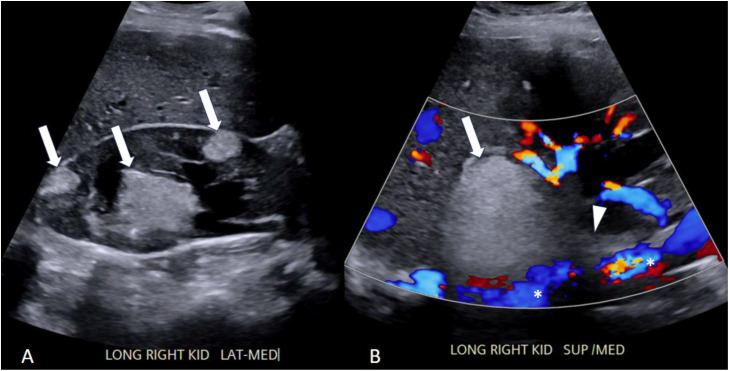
Ultrasound of the right kidney. Grayscale image in the longitudinal plane (A) shows multiple well-circumscribed hyperechoic lesions (white arrows), consistent with fat-containing masses. Color flow doppler image of the right kidney (B) shows no significant blood flow in the lesion (white arrow). The lesion appears to extend medially (arrowhead) to the IVC (*).
